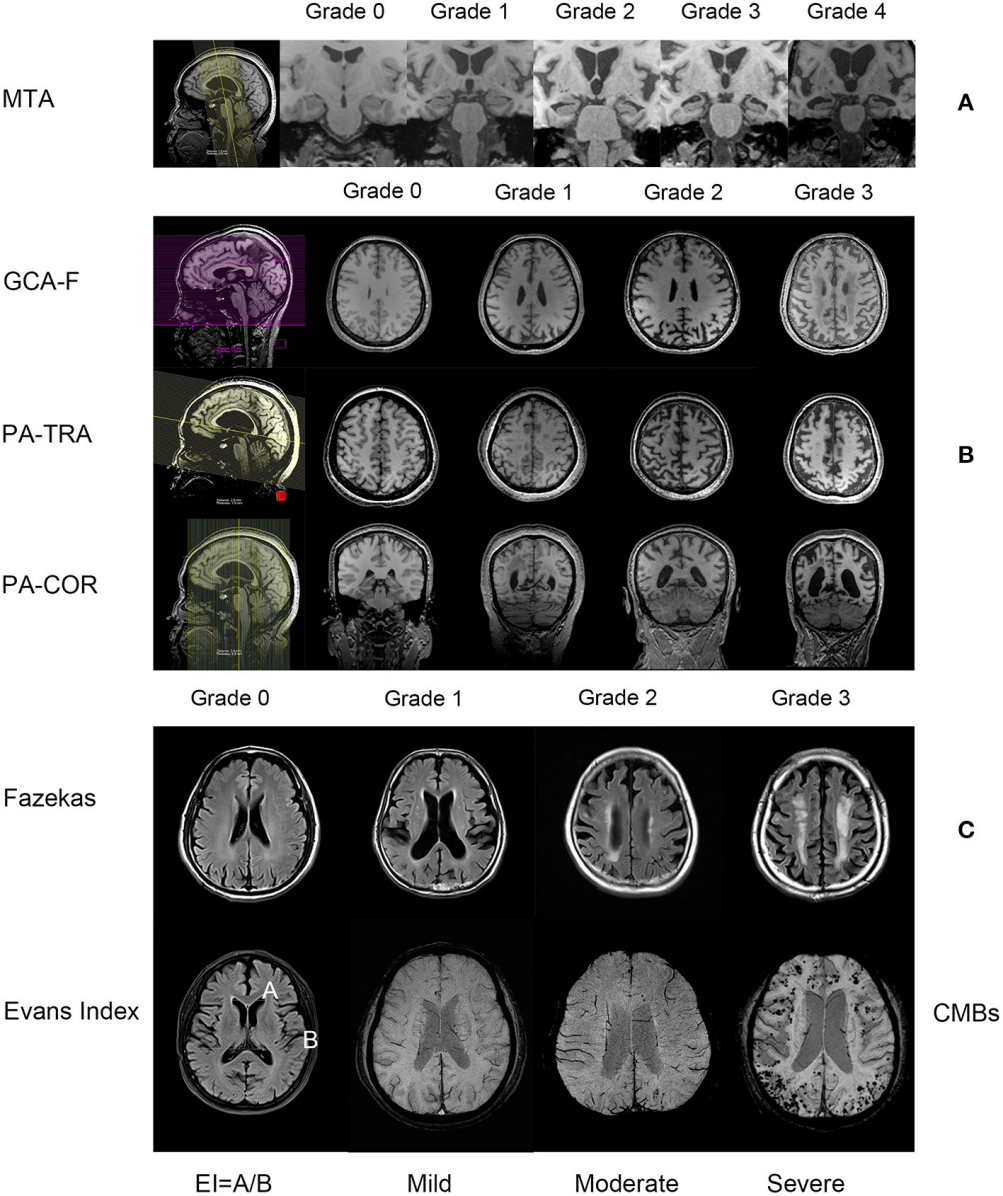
The reconstruction mode and the description of the degree of the MRI visual rating scales (all images are from subjects in this study). (A) contains the MTA visual rating scale, from left to right are the positioning phase, grade 0–4 (3D T1-weighted images, oblique coronal); (B) contains the GCA-F, PA visual rating scale, from left to right are the positioning phase, grade 0–3 (GCA-F is the transverse section of T1-weighted images, PA is the transverse section and coronal section of T1-weighted images); (C) contains the Fazekas visual rating scale, from left to right are grade 0–3 (T2-weighted fluid-attenuated inversion-recovery sequence), the Evans Index, the value is the ratio between the maximal diameter of the anterior horns of the lateral ventricles-A and the greatest internal diameter of the skull-B, and CMBs, according to the number of microbleeds, from left to right are mild, moderate and severe (the figure shows the susceptibility-weighted imaging sequence, the 3D T1-weighted gradient-echo sequence is not shown). MTA, medial temporal lobe atrophy; GCA-F, global cortical atrophy-frontal subscale; PA, posterior atrophy; CMBS, cerebral microbleeds.
